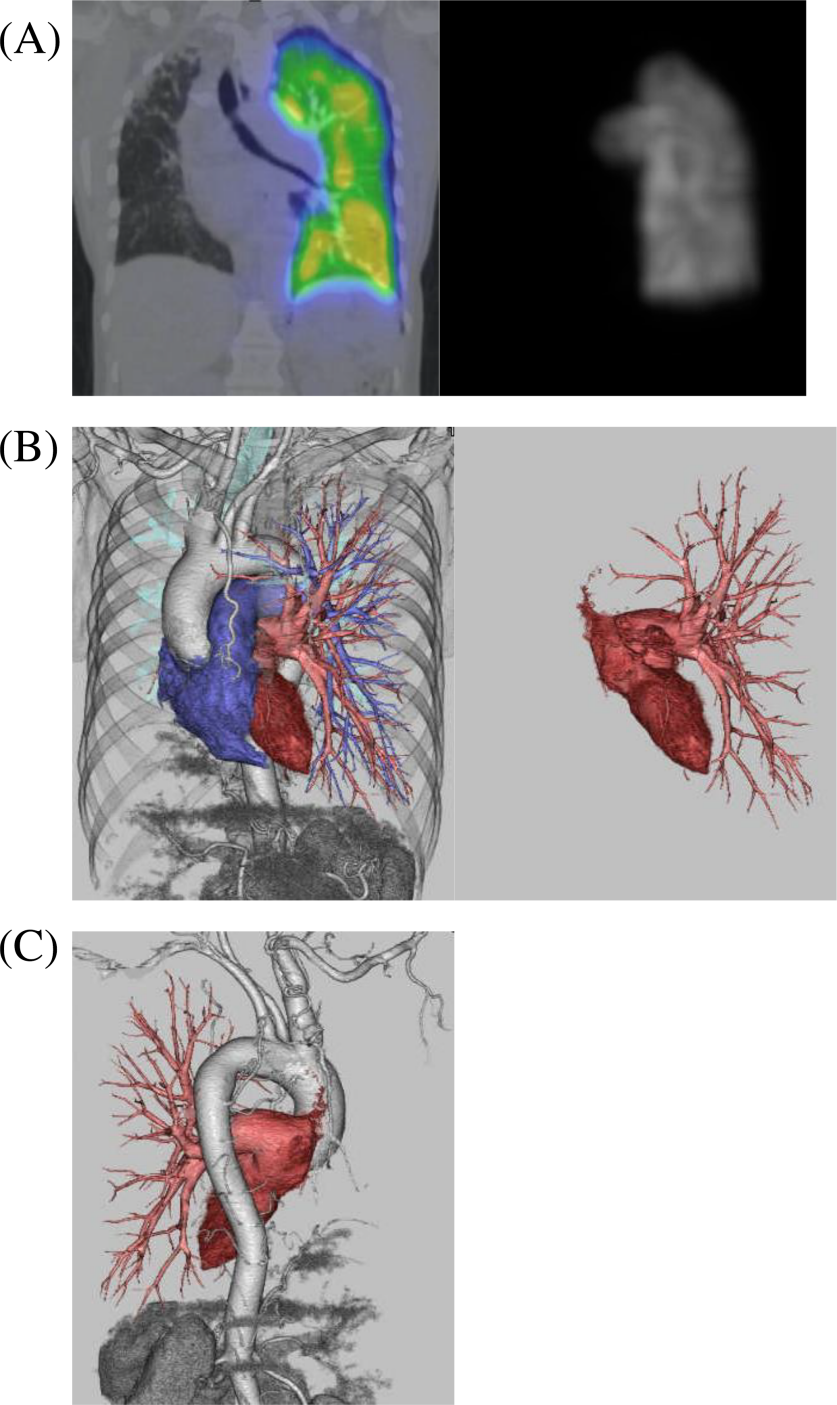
(A) Pulmonary blood flow scintigraphy shows no accumulation in the right lung. (B) Contrast‐enhanced computed tomography images reveal the absence of blood flow from the pulmonary artery in the right lung. (C) Collateral circulation from the bronchial artery to the right lung was observed.
